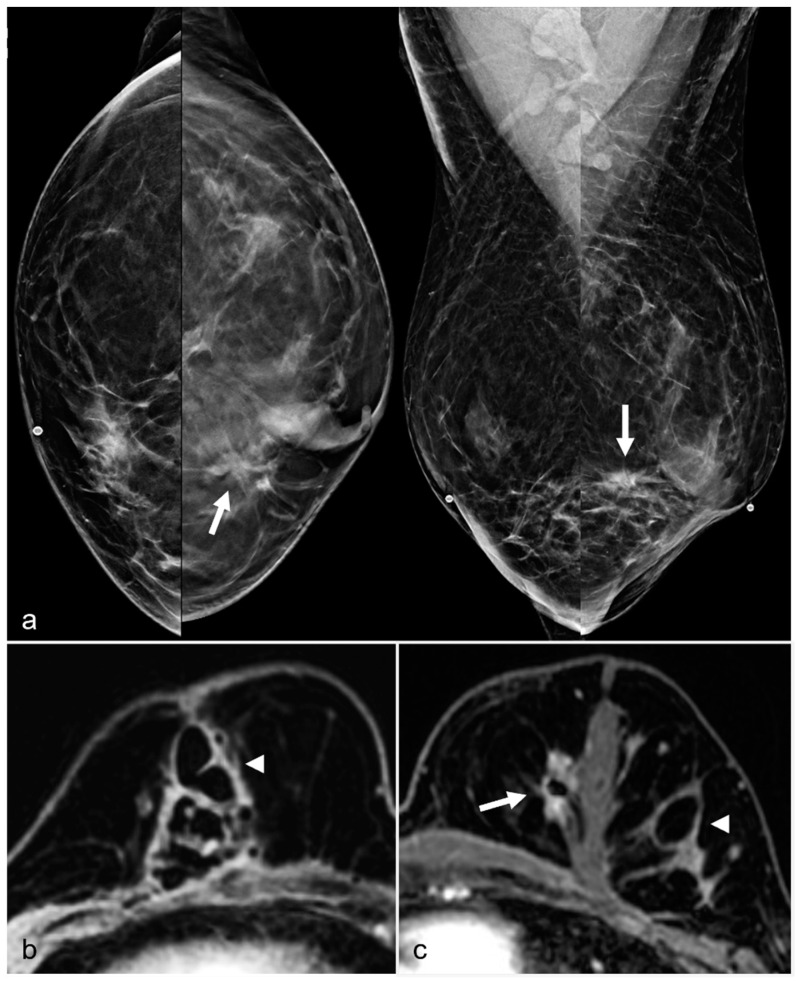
A 52-year-old woman with a history of subglandular silicone implants. Prior mammograms with implant displaced views were negative. A screening mammogram with bilateral CC and MLO views one year after BES (a) demonstrates a spiculated mass in the left lower inner quadrant (arrow), which was biopsied under ultrasound guidance with pathology revealing invasive ductal carcinoma. T1-weighted post-contrast MRI axial images demonstrate areas of fat necrosis in both breasts ((b,c), arrowheads). An enhancing mass with a biopsy clip is seen adjacent to the surgical scar ((c), arrow) in the left breast representing the biopsy proven carcinoma.
